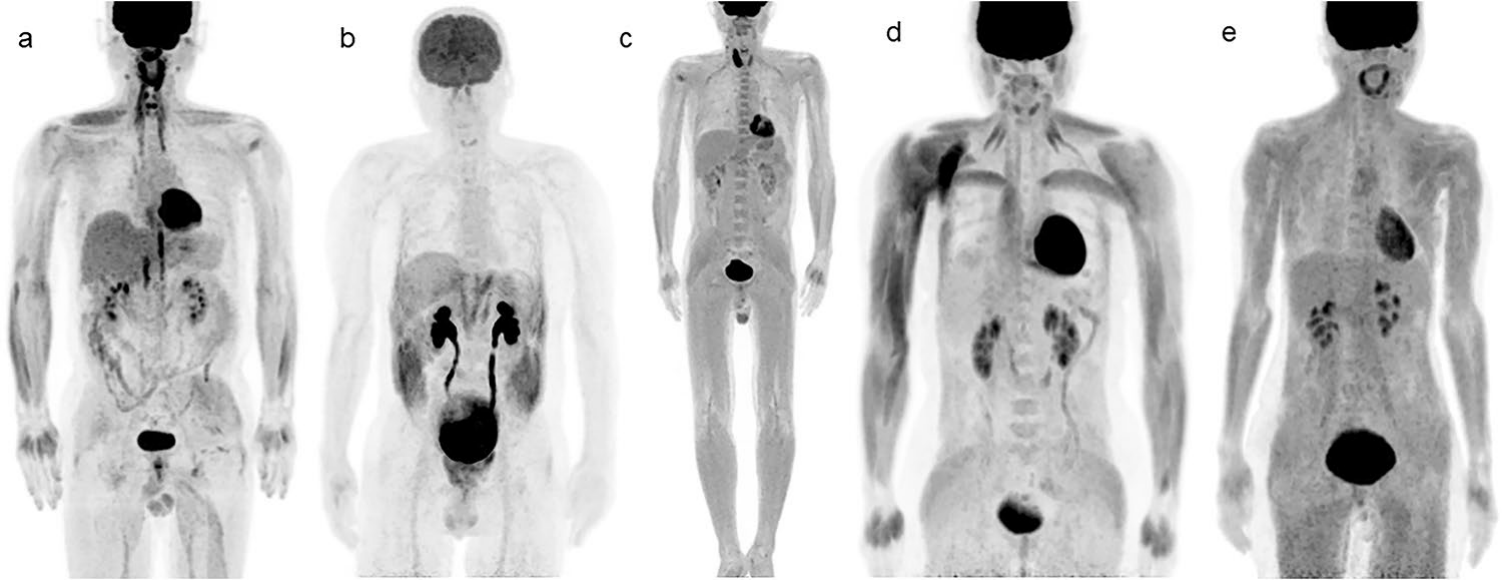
a Stress exercise before FDG injection. b Patient strained during a bowel movement. c Patients administered rapid-acting insulin before the injection of FDG. d Patient diagnosed with insulinoma, and e Patient diagnosed with Basedow’s disease. Abnormal FDG uptake in large muscles is confirmed in these specific situations
